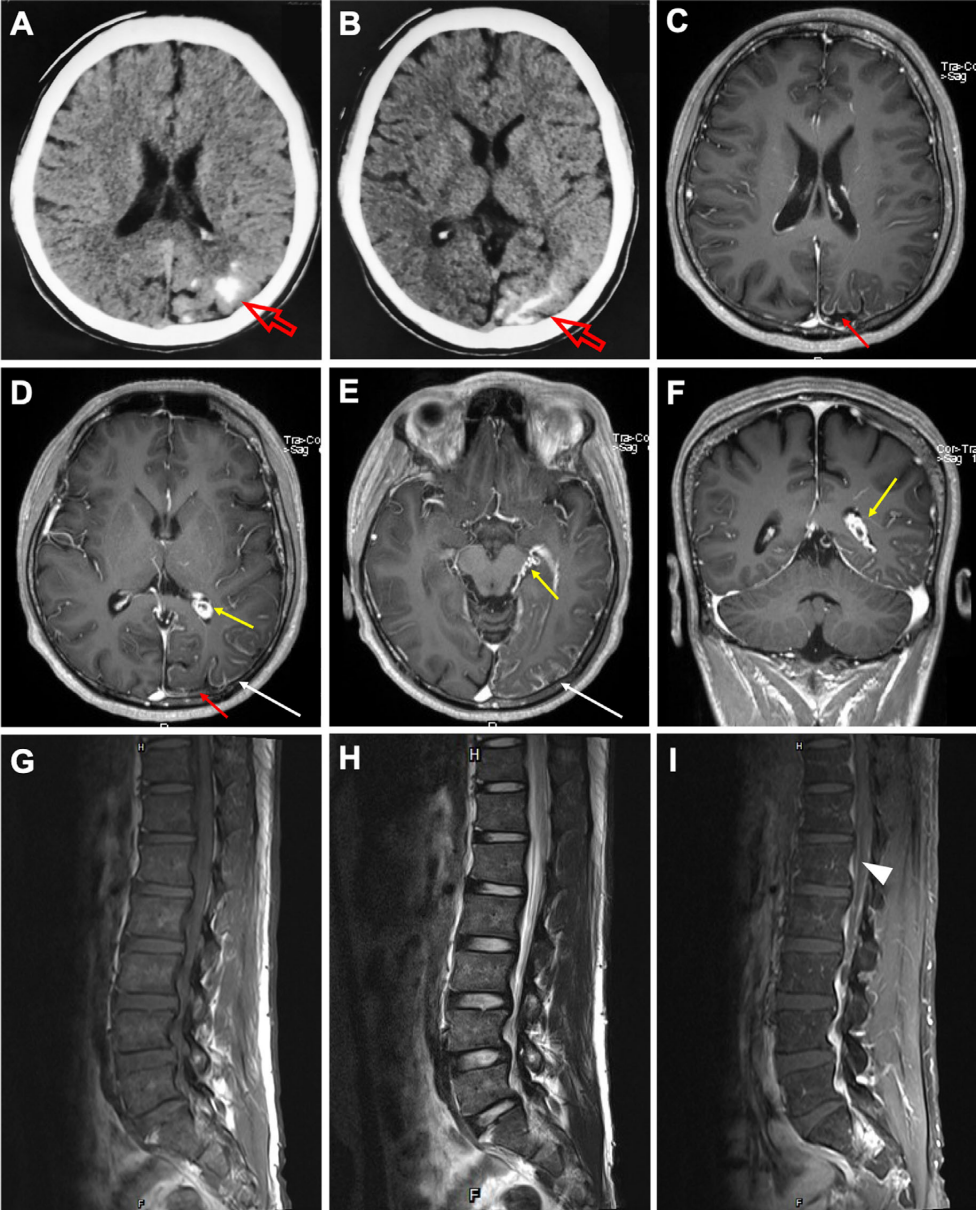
Representative images of brain from the patient with Sturge–Weber syndrome and Klippel–Trenaunay syndrome. Computed tomography scan showed the calcification in the left occipital lobe of the patient (open red arrows) (A, B). T1‐weighted post‐contrast magnetic resonance imaging (MRI) axial view showed left‐sided hemispheric leptomeningeal enhancement (white arrows), left hemispheric brain atrophy predominantly involving left occipital lobe (red arrows), and an enlarged and enhancing left‐sided choroid plexus (yellow arrows) (C–E). T1‐weighted post‐contrast MRI coronal view showed left‐sided choroid plexus enhancement (F). T1‐weighted and T2‐weighted MRI showed lumbosacral segments of spinal cord (G, H). T1‐weighted post‐contrast MRI showed the incrassation and significant enhancement of spinal meninges of lumbosacral segments (I) (arrowhead).
